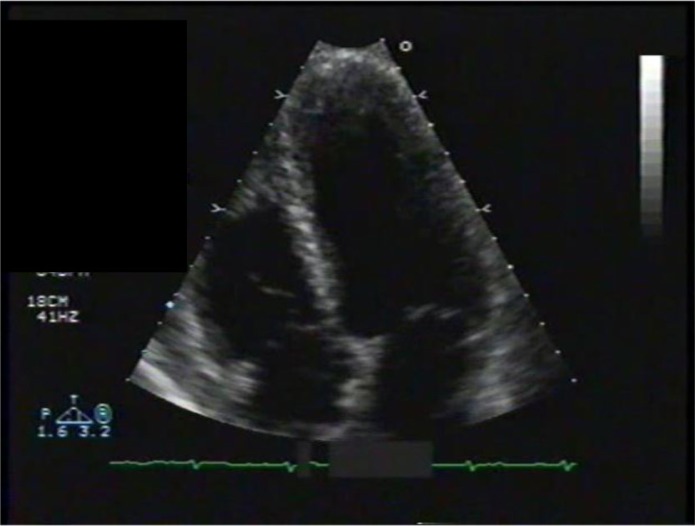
Echocardiogram, post-diastolic.
